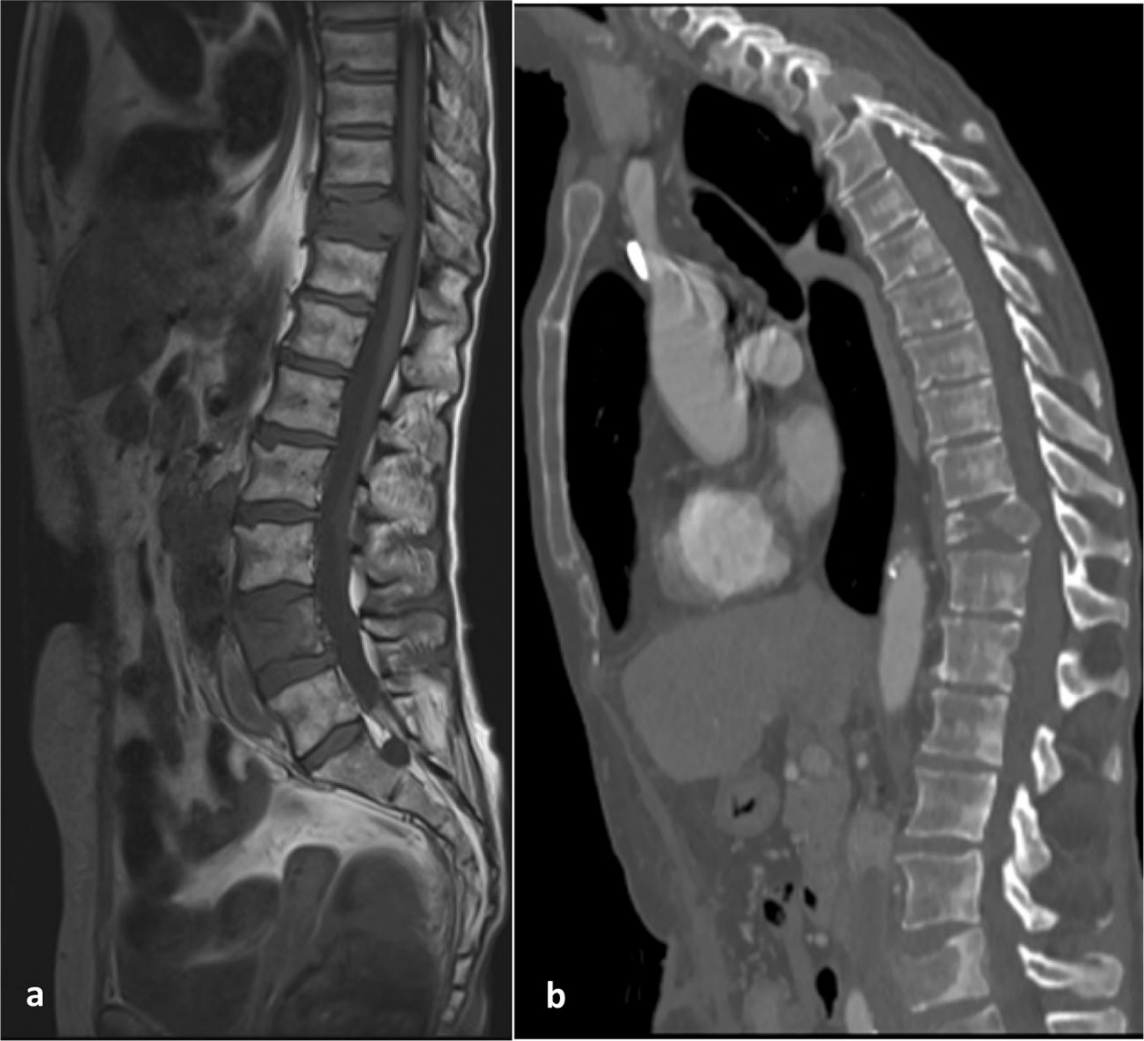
Sagittal T1 weighted MRI (a) and CT (b) images of the thoracolumbar spine demonstrating metastatic infiltration at L4 and T12 with an associated pathological fracture and narrowing of the central vertebral canal
